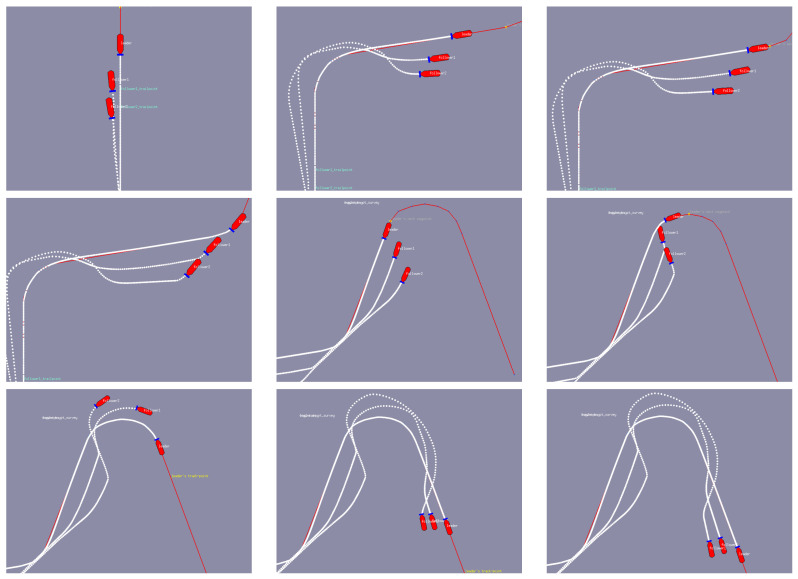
Example of NSCSUV-controlled vehicle behaviour on trajectory no. 2.
